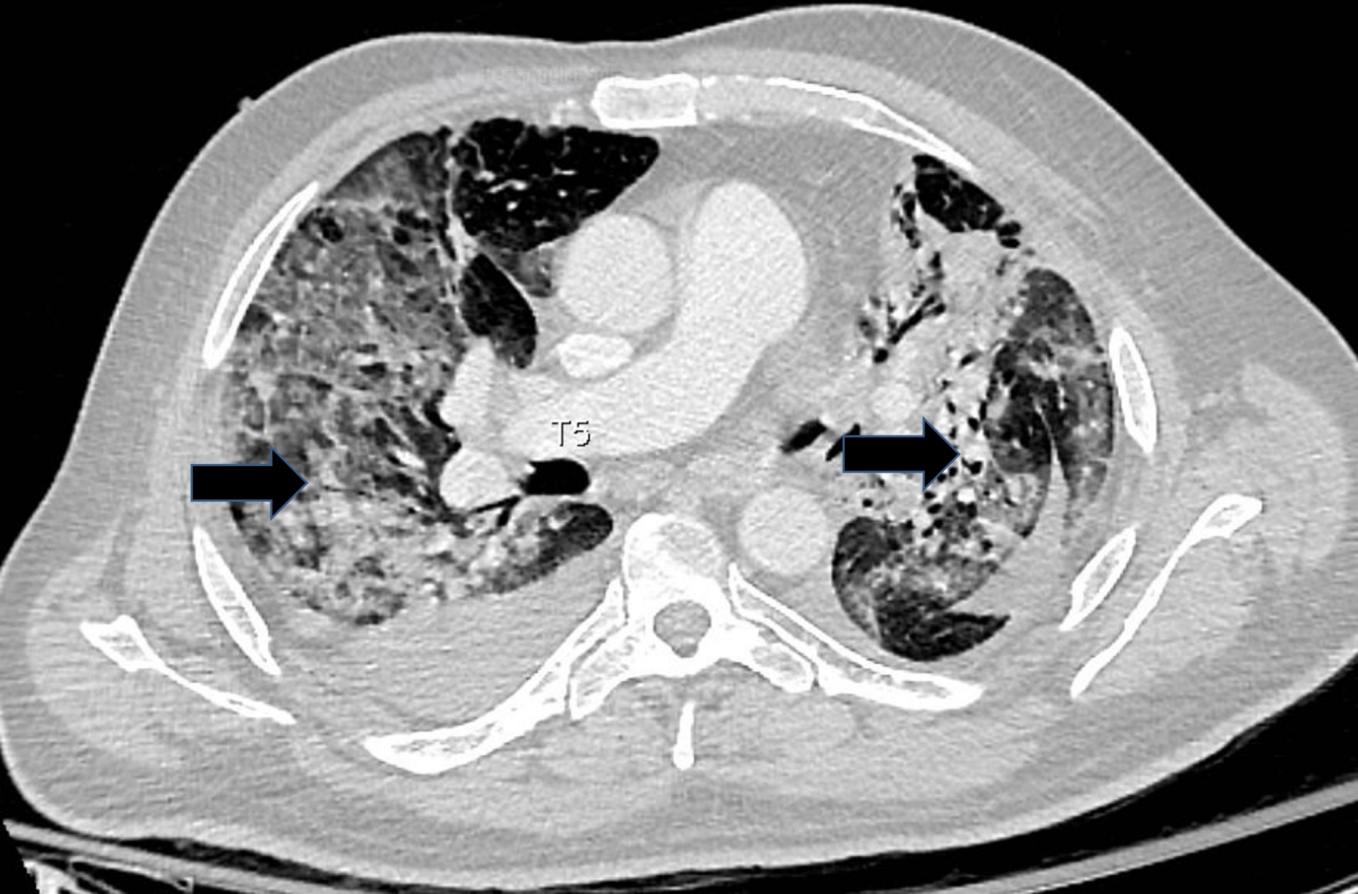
Computed Tomography of the Chest (Axial View). Bilateral diffuse areas of ground-glass opacities involving the majority of the right and left lung consistent with interstitial pneumonitis.
